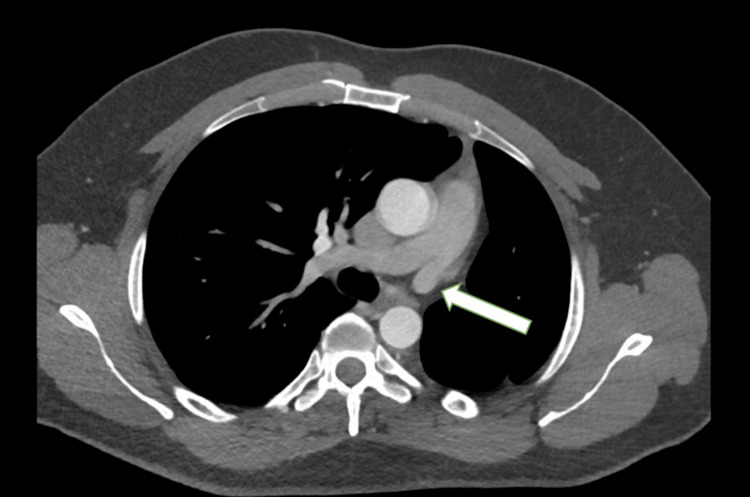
CT chest (mediastinal window) showing a hypoplastic left pulmonary artery (arrow).
